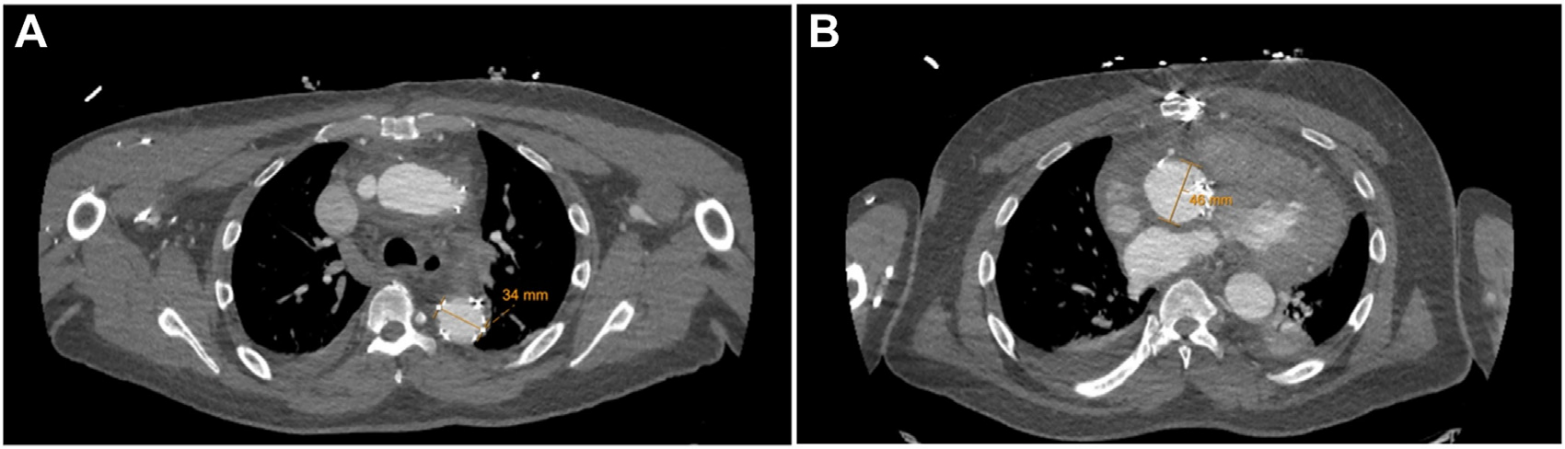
Postoperative contrast-enhanced computed tomography angiography showing measurements of (A) distal arch and (B) aortic root in axial view.
